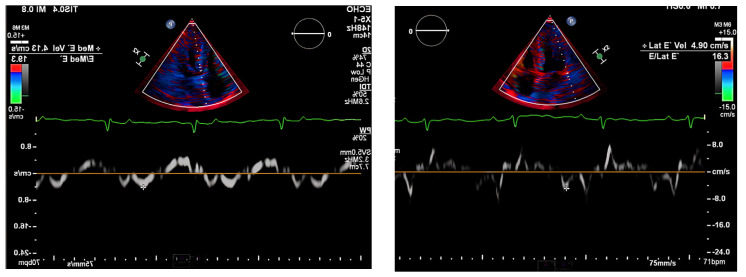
Pulse-wave Tissue Doppler ECHO showing elevated E/E′ ratio and low mitral annulus velocities suggestive of diastolic dysfunction.
